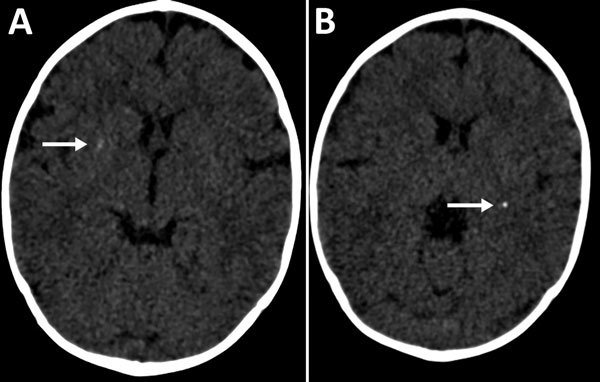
Cerebral computed tomography images of infant with probable congenital Zika virus infection at 7 months of age, Brazil. A) Mild calcification at the right lenticular nucleus (arrow); B) calcifications at the posterior arm of the left internal capsule (arrow).
